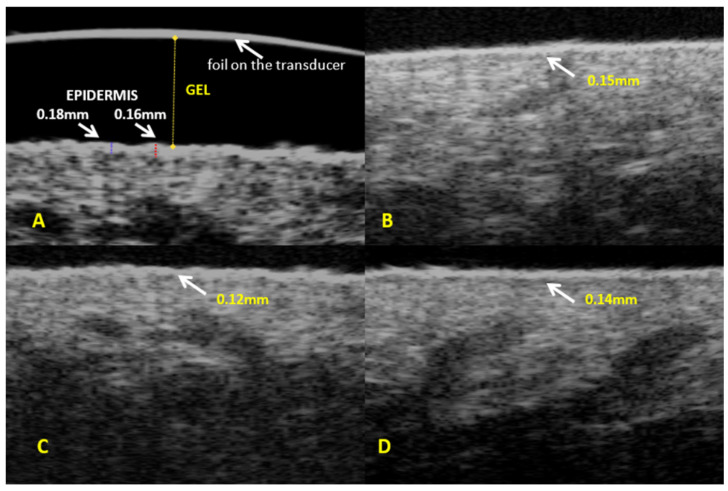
Ultrasound assessment of epidermis—(A). Epidermis thickness: (B)—group 1, (C)—group 2, (D)—group 3.
